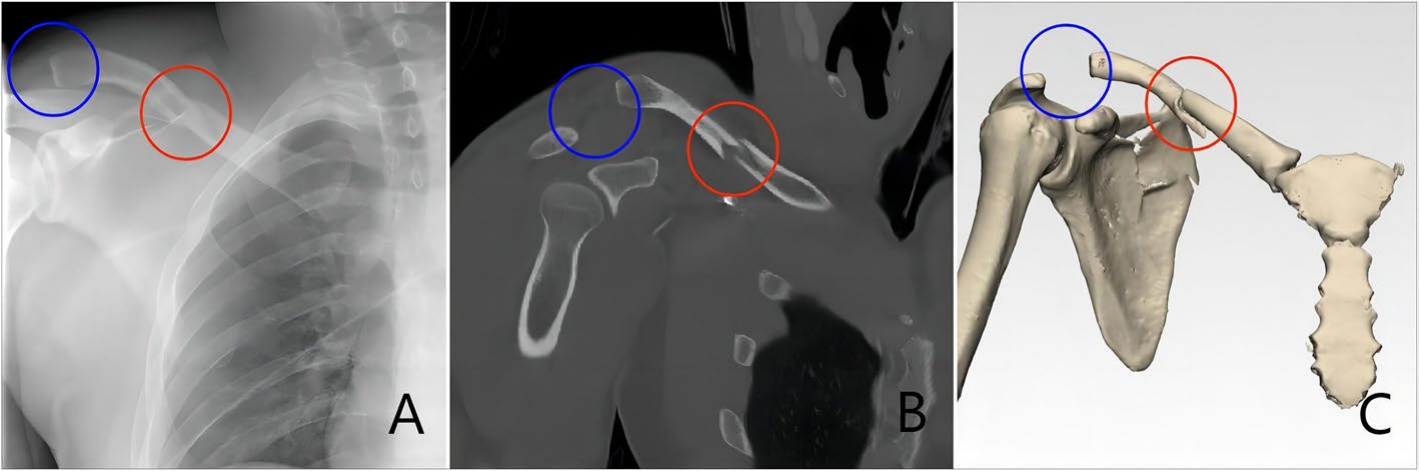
Typical radiologic findings in midshaft fractures with associated ipsilateral acromioclavicular joint injuries. (A) AP view. (B) Coronal section of CT scan; (C) 3D CT reconstruction. Blue circle, type V acromioclavicular joint dislocation; red circle, displaced midshaft clavicle fracture
